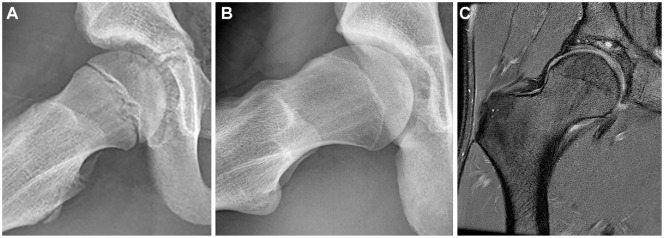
Example of a participant at (A) baseline, (B) 5-year follow-up, and (C) 12-year follow-up. (A) A frog-leg lateral radiograph of the right hip (aged 12 years) showing a spherical femoral head. (B) Presence of cam morphology at 5-year follow-up (aged 17 years). (C) Coronal proton density–weighted fat-suppressed magnetic resonance imaging of the same right hip at 12-year follow-up (aged 24 years) showing cam morphology and a local cartilage defect at the superomedial part of the acetabulum with an adjacent subchondral cyst.
